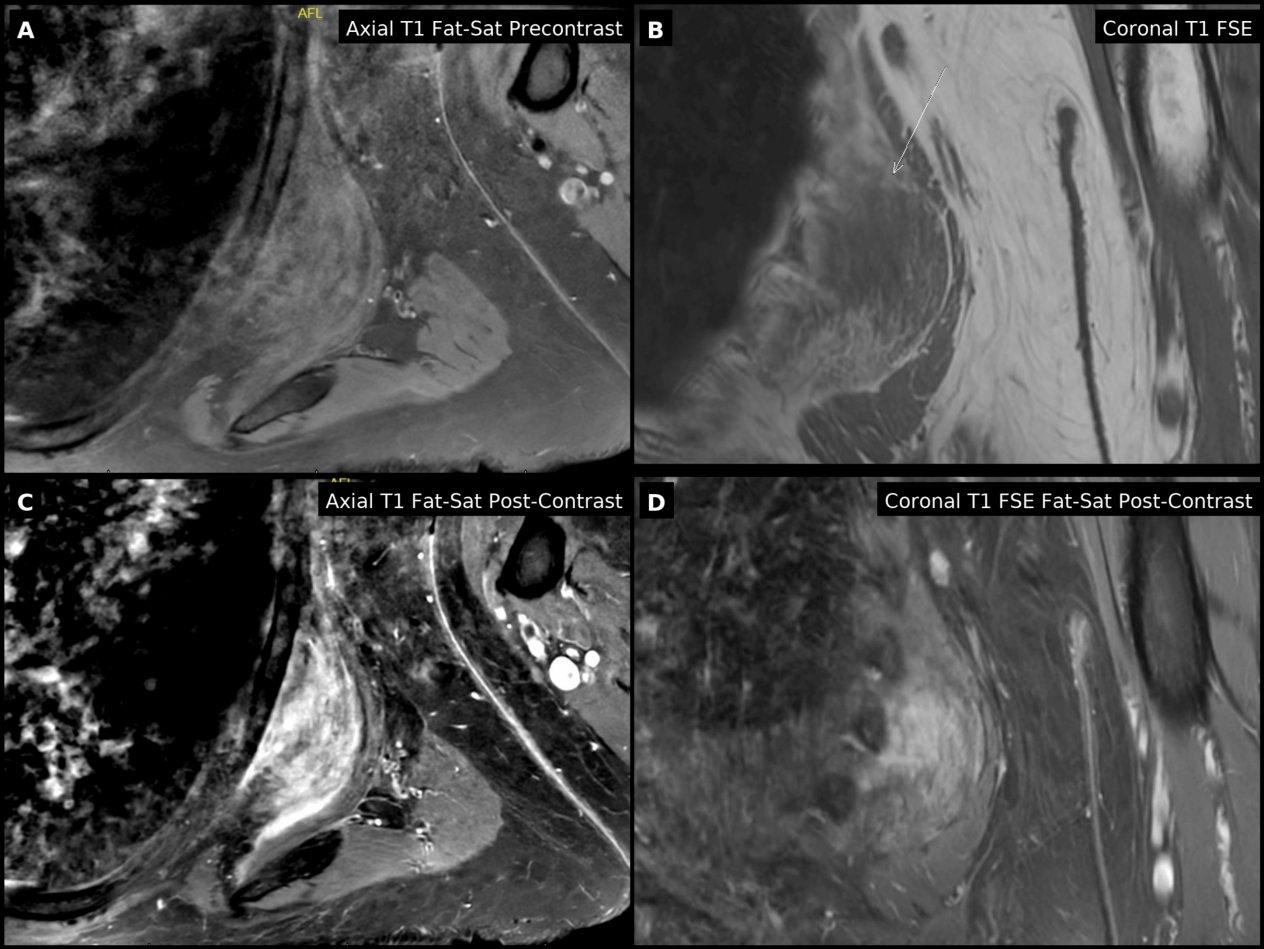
Pre-procedure MRI of elastofibroma dorsi (A) Axial T1 fat-saturated pre-contrast image showing a well-defined, striated soft-tissue mass deep to the left scapula, consistent with elastofibroma. (B) Coronal T1 FSE image demonstrating the lesion (arrow) between the latissimus dorsi and serratus anterior muscles. (C) Axial T1 fat-saturated post-contrast image showing mild peripheral enhancement. (D) Coronal T1 FSE fat-saturated post-contrast image confirming the characteristic alternating fibrous and fatty pattern.
